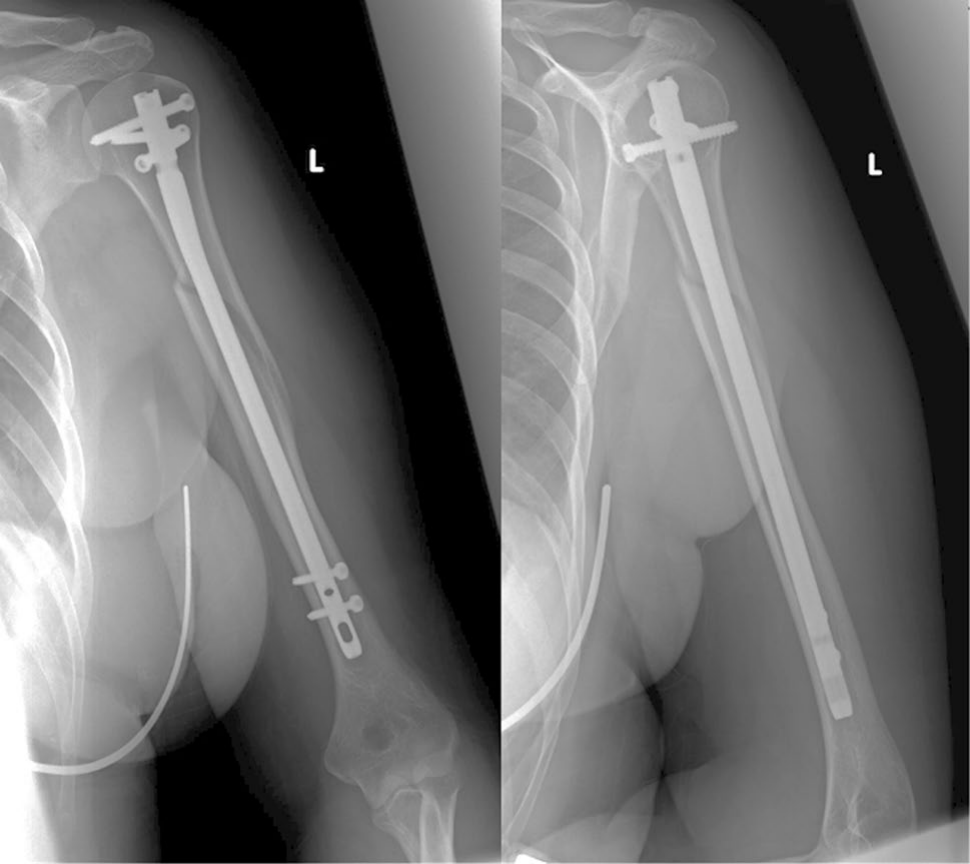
postoperative a.p. and lateral radiograph showing lateral–medial locking technique
